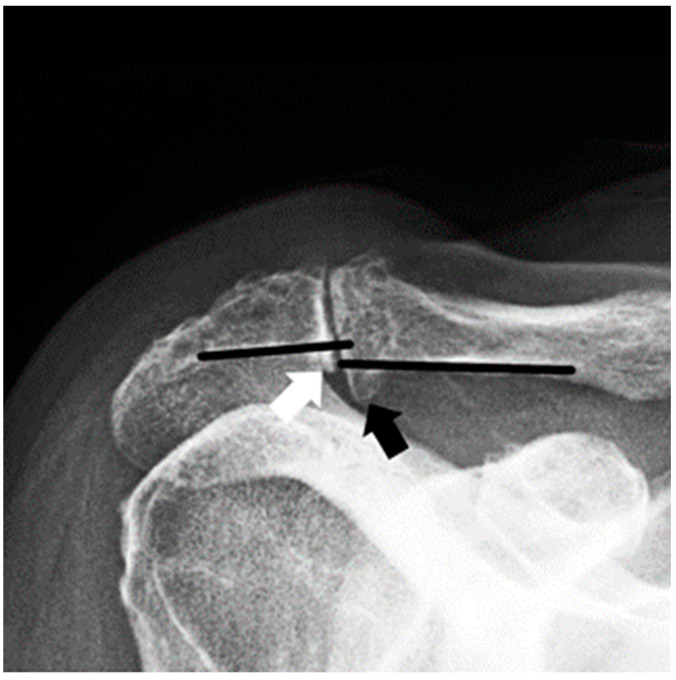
Zanca view radiograph of a right shoulder. The black arrow shows an inferior bony spur of the distal clavicle, the white arrow shows a small inferior bony spur of the medial acromion.
